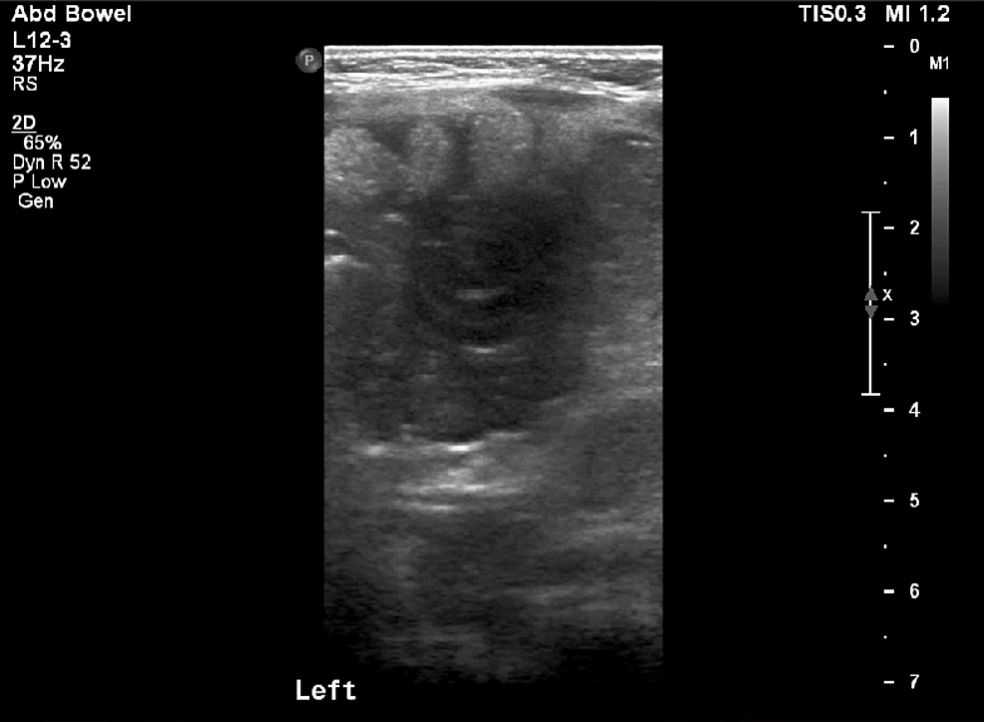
Abdominal ultrasound demonstrates “target sign” suggestive of intussusception.
